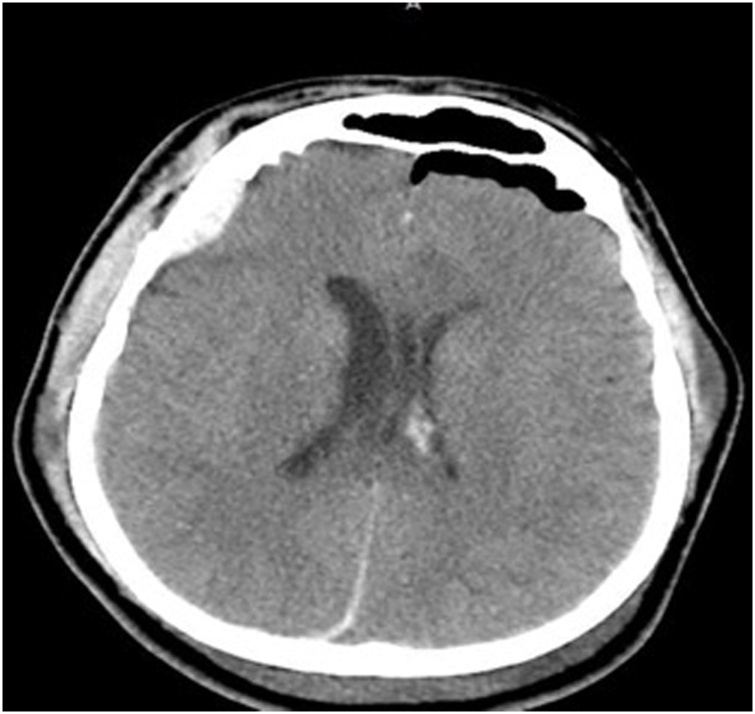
CT scan of head on day 3 showing progressive ventricular widening and frontal pneumocephalus.
